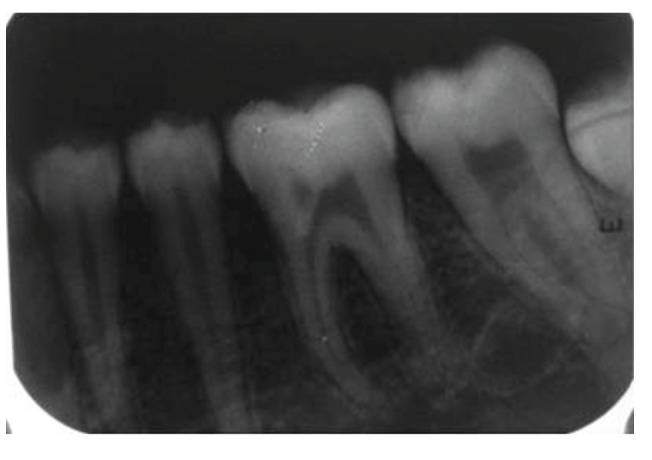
Intraoral periapical radiograph with 36 at 2-year followup. No significant pulpal or periapical changes.
